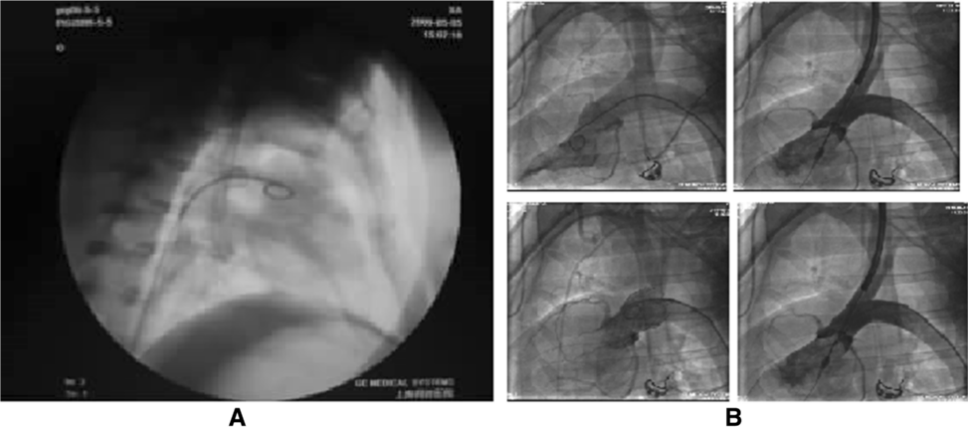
Radiography of valved stent. The radiographs of aortic valved stent in the ascending aorta (A) and during implantation (B).
